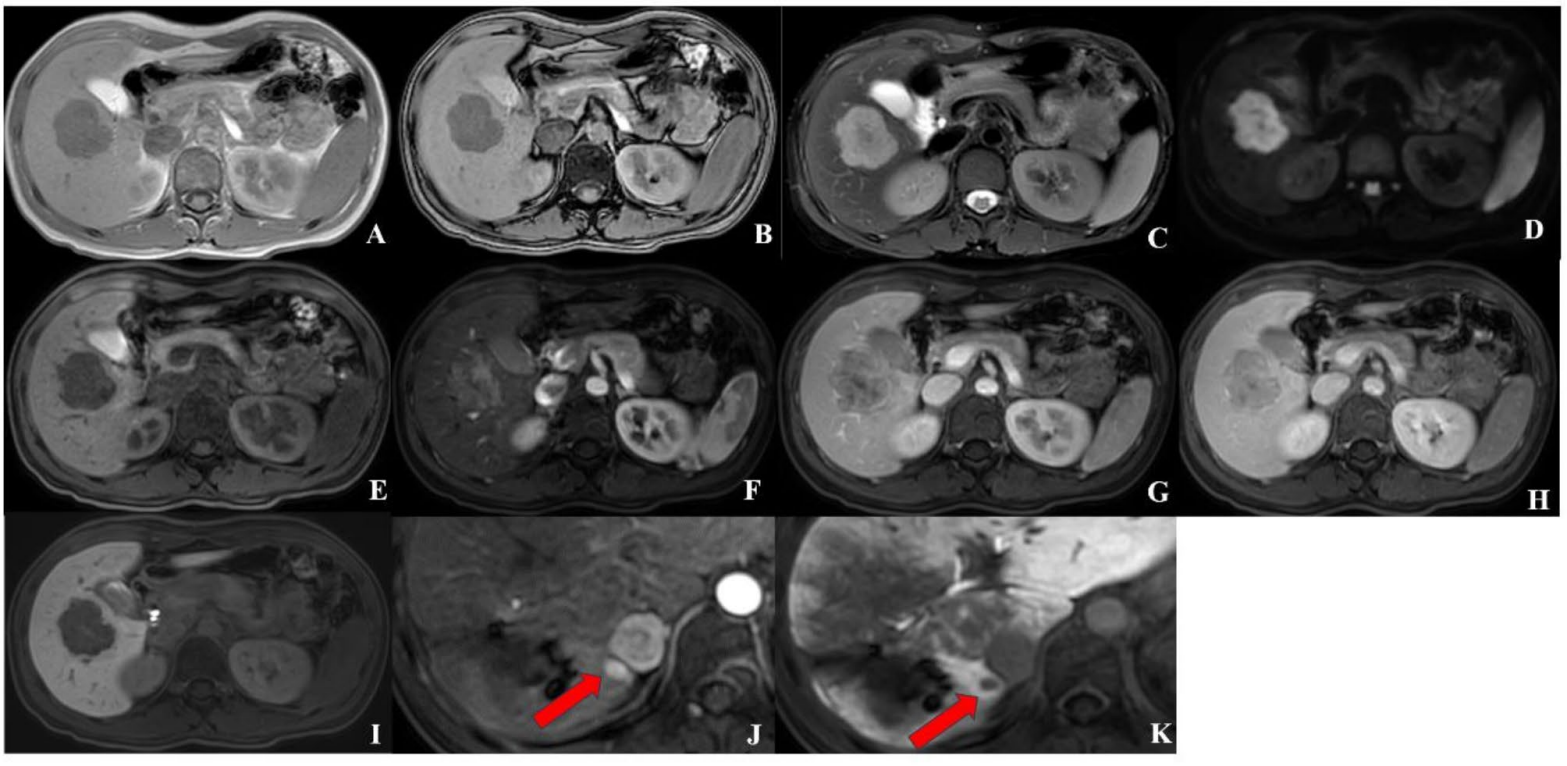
26-year-old woman with surgically proven HCC in segment V. (A-B) T1-weighted images in phase and out phase showing hypointensity; (C) T2-weighted images showing hyperintensity; (D) DWI showing hyperintensity; (E)Pre-contrast T1-weighted images showing hypointensity; (F) Arterial phase images showing heterogeneous arterial hyperenhancement (APHE) with infiltrative appearance; (G) Portal venous phase showing nonperipheral washout; (H) Transitional phase showing hypointensity; (I) Hepatobiliary phase showing targetoid appearance of peripheral low signal and high signal in the center; (J-K) A new nodule with diameter of 8 mm in segment VI-VII after surgery showing APHE on arterial phase and low signal on hepatobiliary phase (Red arrow)
